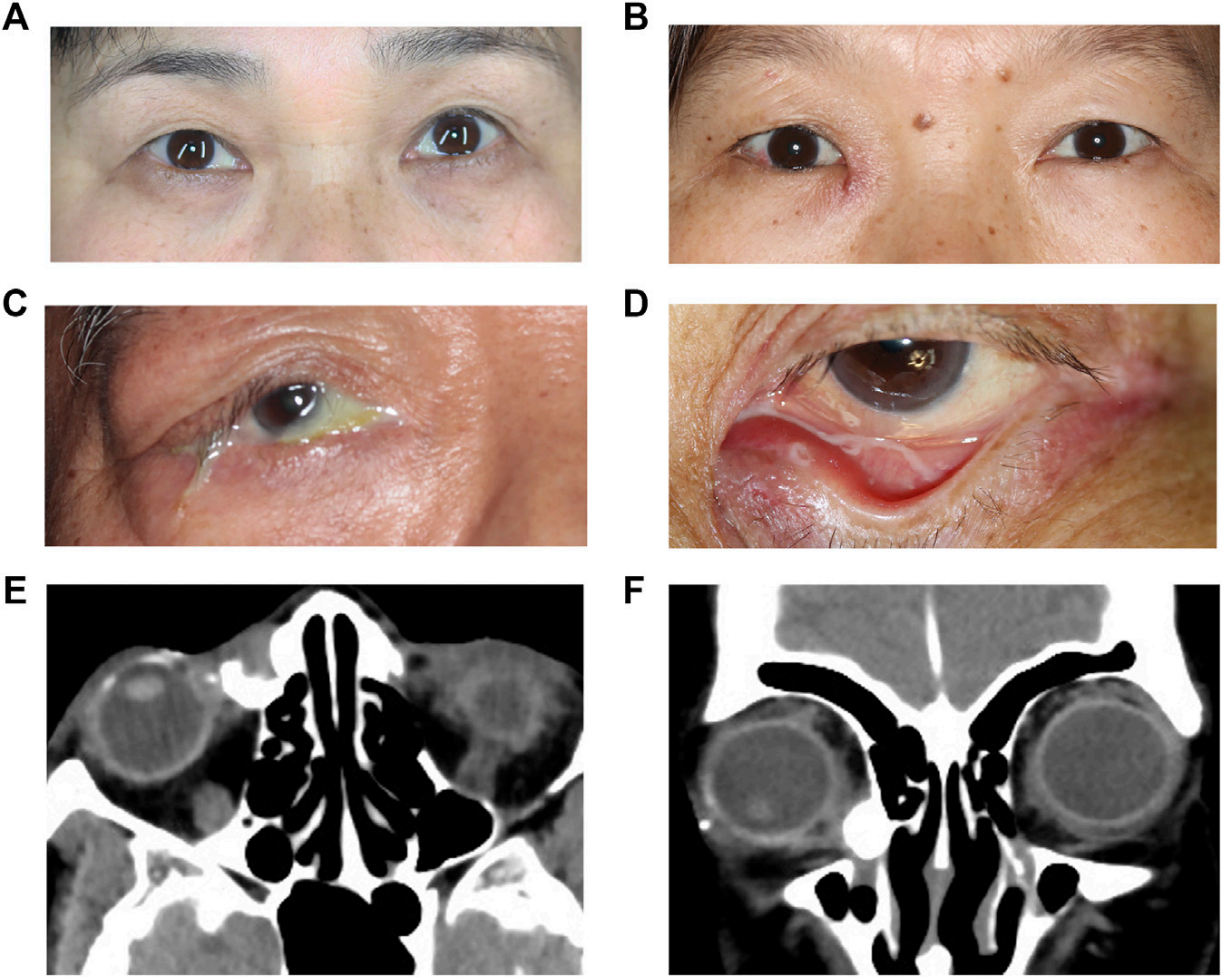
Clinical manifestation of chronic dacryocystitis patients. (A–D) Representative clinical characteristics of patients with chronic dacryocystitis. (E,F) Representative computed tomography-dacryocystography (CT-DCG) images of patients with chronic dacryocystitis.
